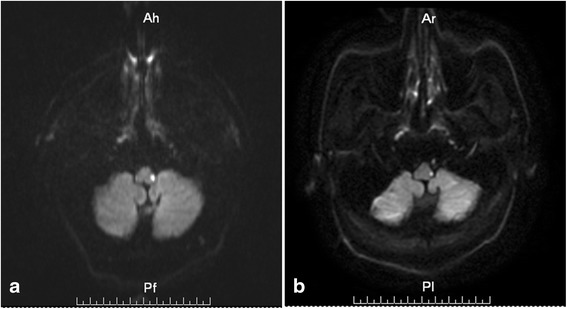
MR dffusion weighted imaging of infarct lesion in the left side of dorsolateral medulla. a DWI shows the left-sided dorsolateral medullary infarction. b The infarct volume became smaller at the ninth day after admission to our hospital
